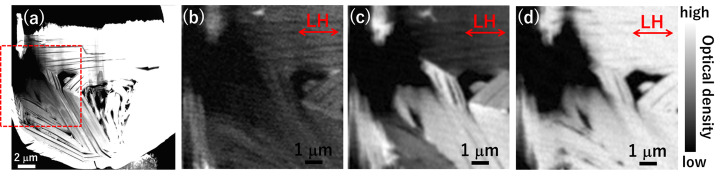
(*a*) TEM (dark field) image of natural spherical graphite. The red dotted line shows the FOV of XA images obtained via cSTXM with a rotation angle of θ = 0°. XA images at θ = 0° (LH) with energies of (*b*) 280.0, (*c*) 285.7, and (*d*) 292.0 eV. The red arrow indicates the X-ray polarization directions of LH.
